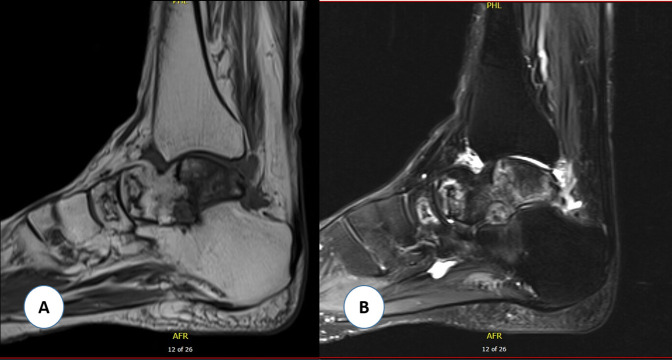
T1-weighted (A) and T2-weighted (B) MRI images of the left ankle demonstrating area of acute bone marrow edema with abnormal signal intensity affecting the talus and navicular bones.
